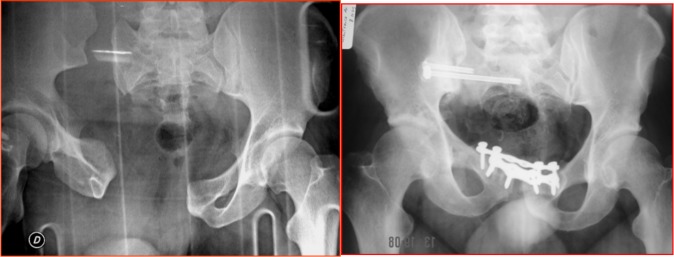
Case of a female patient (42 years old) with right sacroiliac joint instability (C1 Tile injury) and opening of the pubic symphysis following suicidal attempt by fall from height (picture on the left). The patient was initially treated with reduction of the vertical shear and double plate fixation of the pubic symphysis using (anterior approach) and this was followed after 3 days by percutaneous CT-guided screw fixation of the sacroiliac joint (picture on the right).
